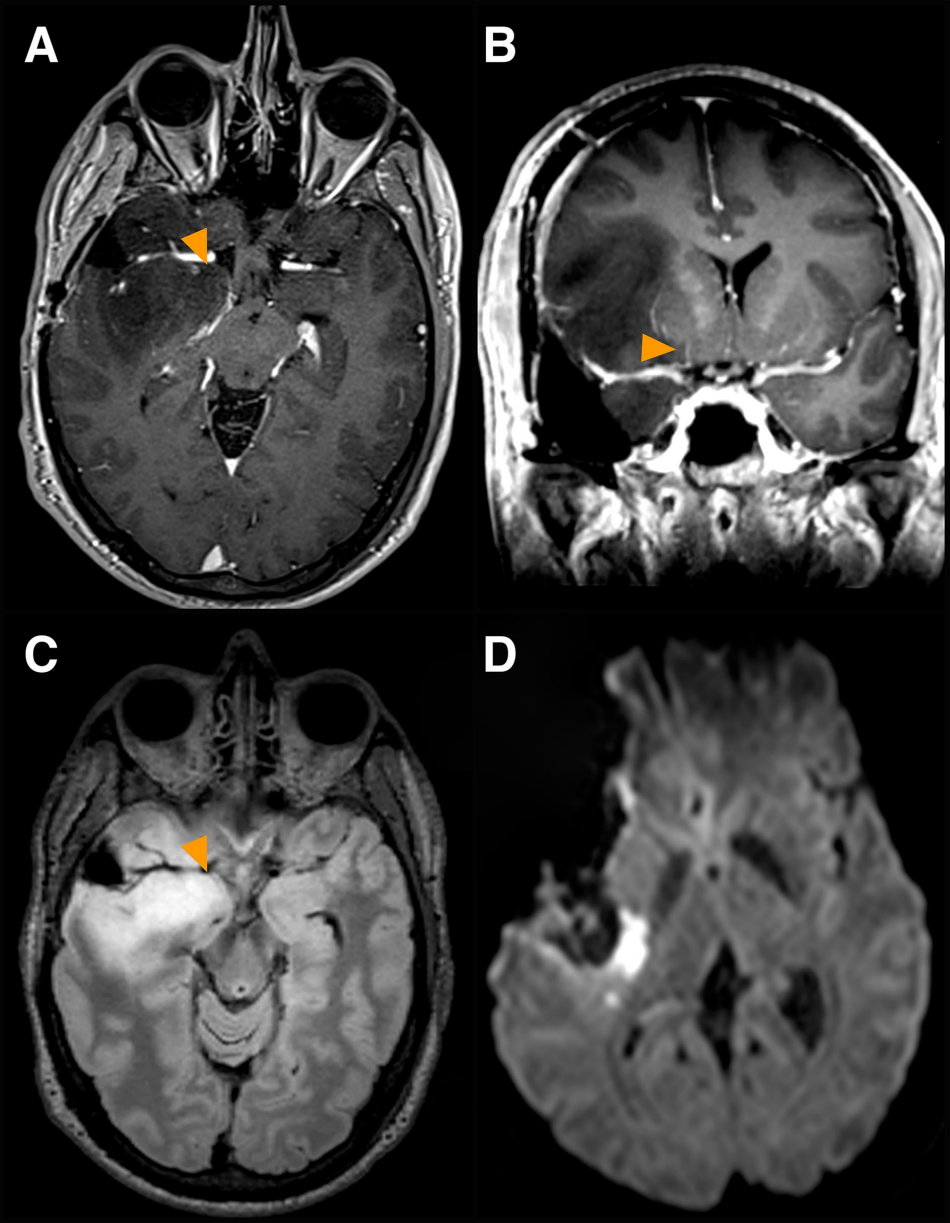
Involvement of other deep perforators. A case of a dominant fronto-tempo-insular low-grade glioma is presented. (A, B) Pre-op axial (A) and coronal (B) volumetric 3T MR T1 post-Gadolinium images displaying tumor involvement of other deep perforators (*yellow arrows*), arterial branches originating at the origin of the anterior choroidal artery from the internal carotid artery, medial to the LSA complex arteries. (C) corresponding pre-op axial FLAIR image. (D) post-op DW axial image, displaying ischemia posteriorly to the surgical cavity at the level of the posterior limb of the internal capsule. Severe MEP fluctuations and significant reduction occurred at the end of the resection. SEPs were unchanged. Arterial pressure was increased. The patient woke up with severe upper and lower limb paresis, which recovered progressively and entirely within 3 months after rehabilitation.
